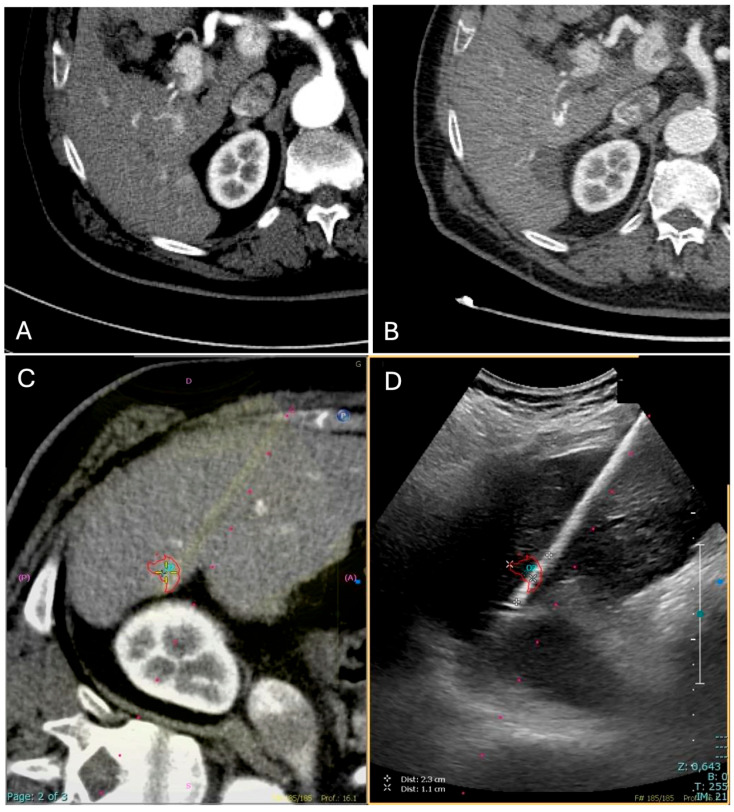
Contrast-enhanced CT (CECT) in the arterial phase demonstrates a newly diagnosed HCC located in segment VI of the liver, near the hepatic capsule. (A) The lesion shows intense arterial-phase hyperenhancement (wash-in), a typical imaging hallmark of HCC. (B) One-month follow-up CECT shows no residual enhancement, consistent with complete response to treatment. (C) CT–US fusion image following co-registration, enabling precise visualization of the lesion (highlighted by a red target). (D) The corresponding real-time US image during the ablation procedure, with the lesion and ablation needle clearly visualized (highlighted by a red target).
